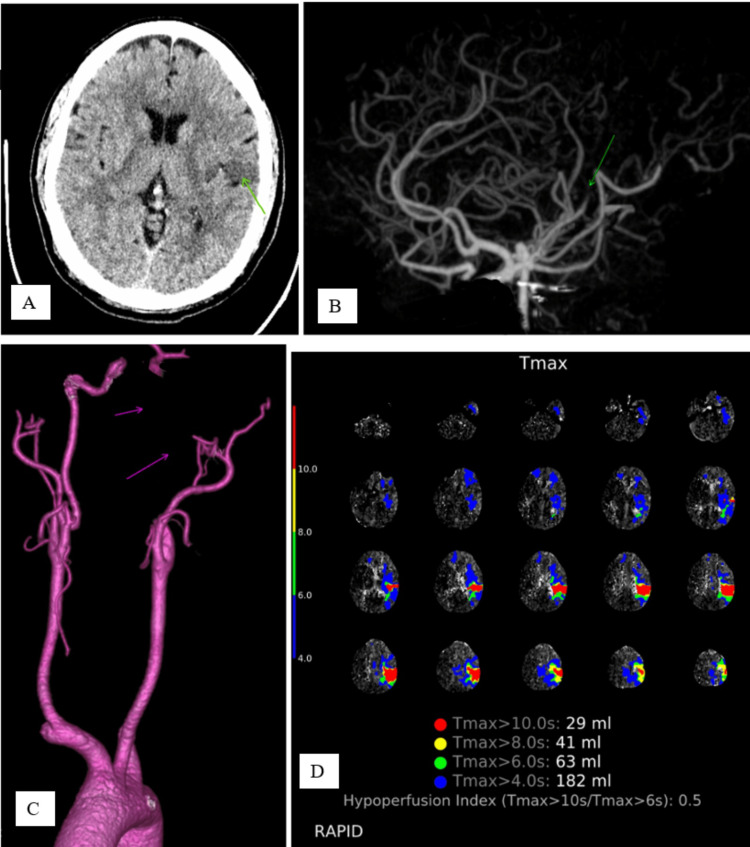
Acute neuroimaging on presentation (A) Non-contrast computed tomography (CT) of the head demonstrating subtle hypodensity with loss of the insular ribbon in the left insular cortex (arrow) and effacement of the adjacent frontal operculum. (B) Intracranial CT angiography maximum-intensity projection demonstrating abrupt cut-off of a distal M2 branch of the left middle cerebral artery (arrow), consistent with occlusion. (C) Three-dimensional volume-rendered CT angiography of the cervical vasculature demonstrating occlusion of the left cervical internal carotid artery (arrows), with reconstitution of flow at the petrous segment, consistent with dissection. (D) RAPID (Rapid Processing of Perfusion and Diffusion; RapidAI, Menlo Park, CA, USA) Tmax perfusion map demonstrating a left middle cerebral artery (MCA)-territory deficit (Tmax > 6 s = 63 mL) with a hypoperfusion index of 0.5. No ischemic core was identified on the corresponding cerebral blood flow (CBF) map (not shown), consistent with a favorable target-mismatch profile amenable to reperfusion therapy.
